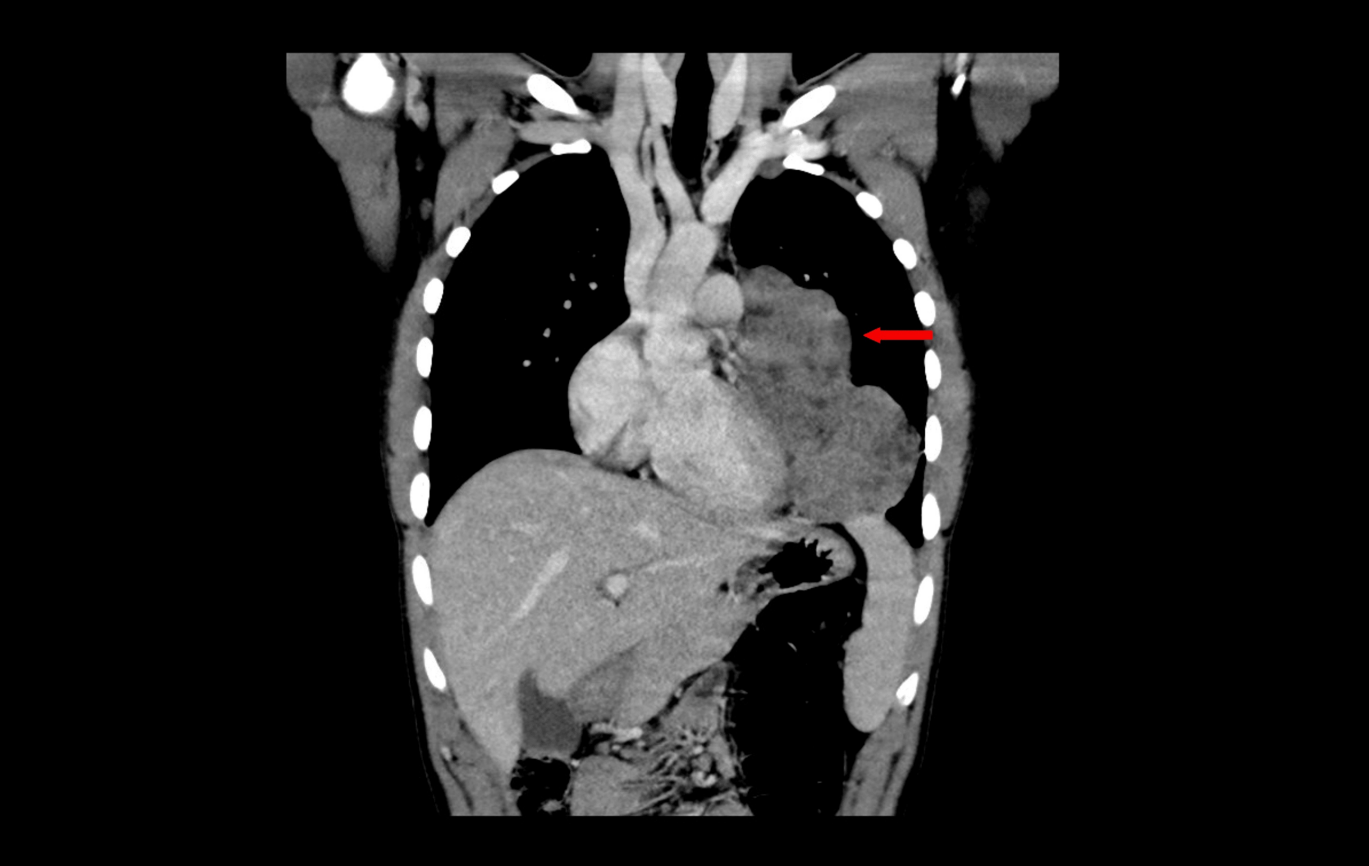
Coronal view of a computed tomography chest with intravenous contrast showing a large anterior mediastinal mass (arrow) draping over the left heart and pericardium extending along the mediastinal and posterior lateral chest wall pleura and lower chest into the left hemithorax.
